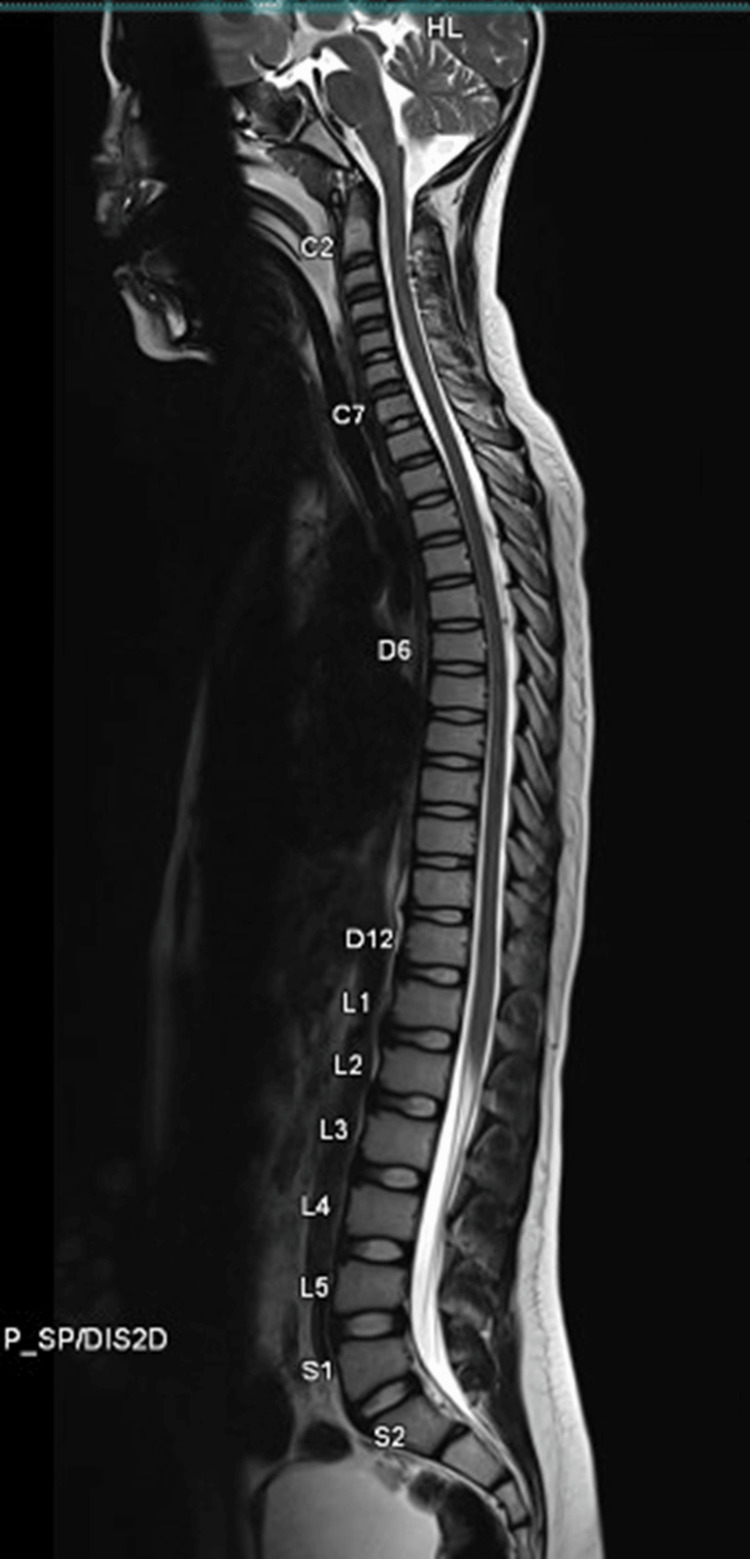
MRI whole spine screening showed no abnormal findings
